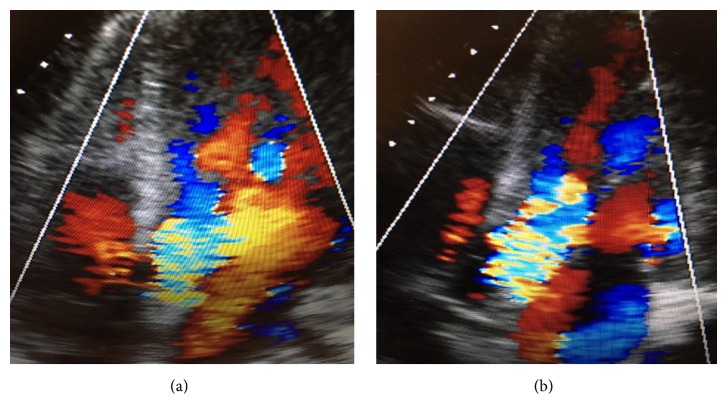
Echocardiogram five-chamber view showing severe aortic regurgitation.
